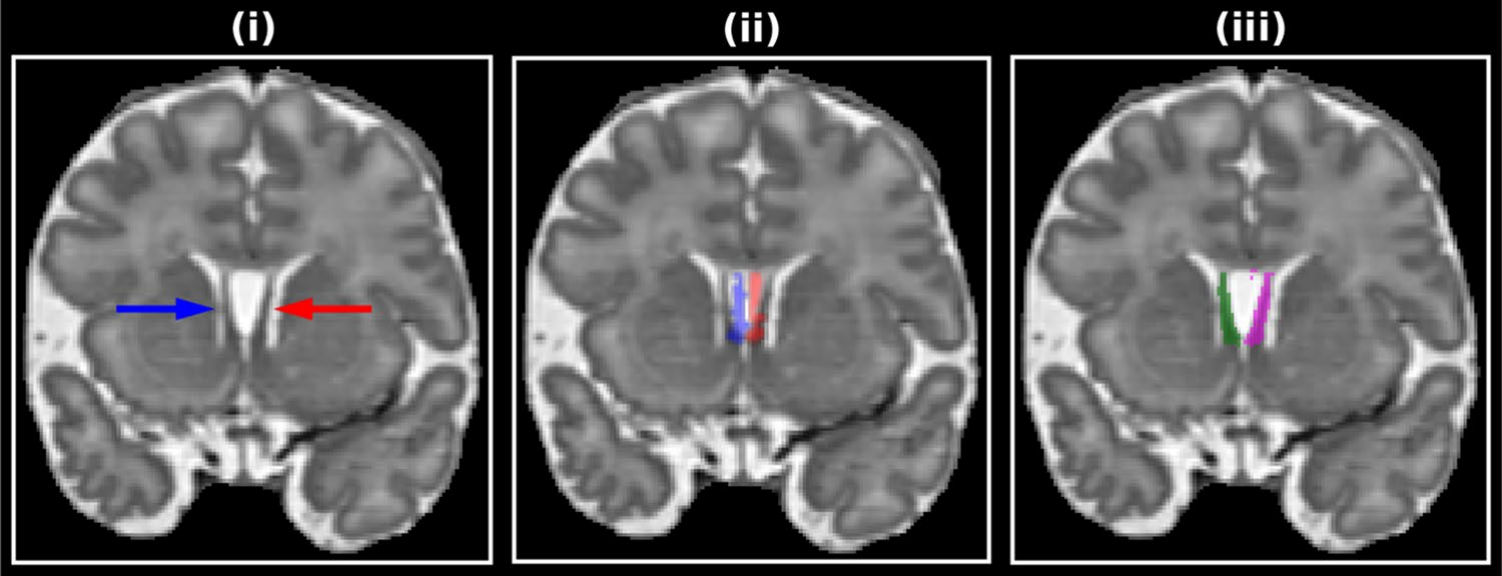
Septum pellucidum (SP) mask generation example. (i) shows the original T2-weighted subject image with the left/right SP denoted by red/blue arrowheads. (ii) shows the template left/right SP masks after registration. (iii) shows the estimated SP masks after lateral search from the masks in (ii)
